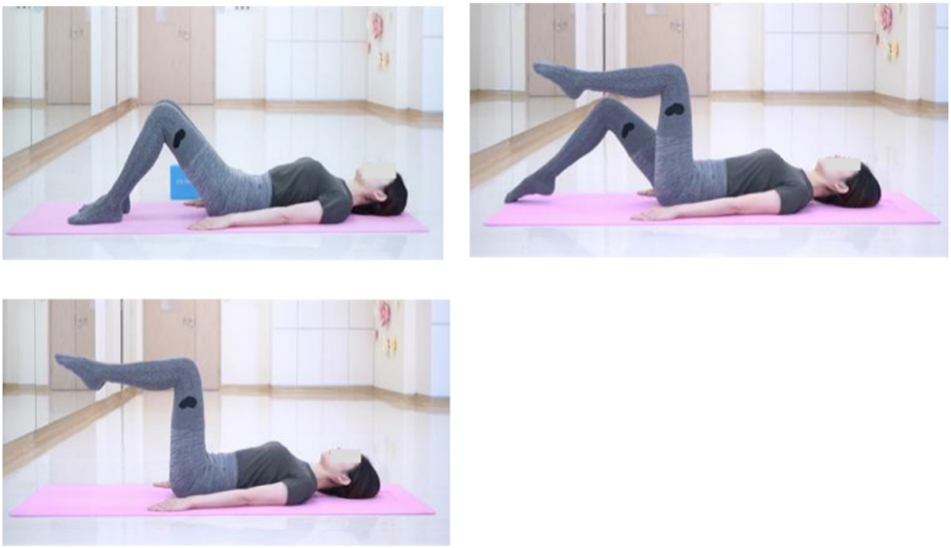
During rehabilitation training, we will provide personalized adjustments to the intensity, difficulty, and duration time based on the physical condition of each parturient under professional guidance, while also paying attention to ongoing evaluation and feedback. Regularly evaluate the IRD and pelvic floor muscle function and monitor progress through ultrasound or electromyography. Adjust the training plan based on the evaluation results to ensure that each mother can maximize the recovery effect under safe conditions.
